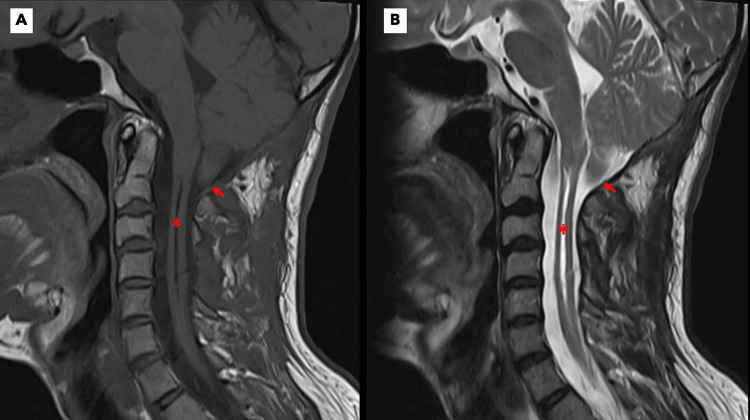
Post-operative cervical MRI findings Sagittal T1 (A) and T2 (B) weighted MRI demonstrate a marked reduction in the volume of the previously noted syrinx (stars), with the persistence of tonsillar herniation (arrows). MRI: magnetic resonance imaging
